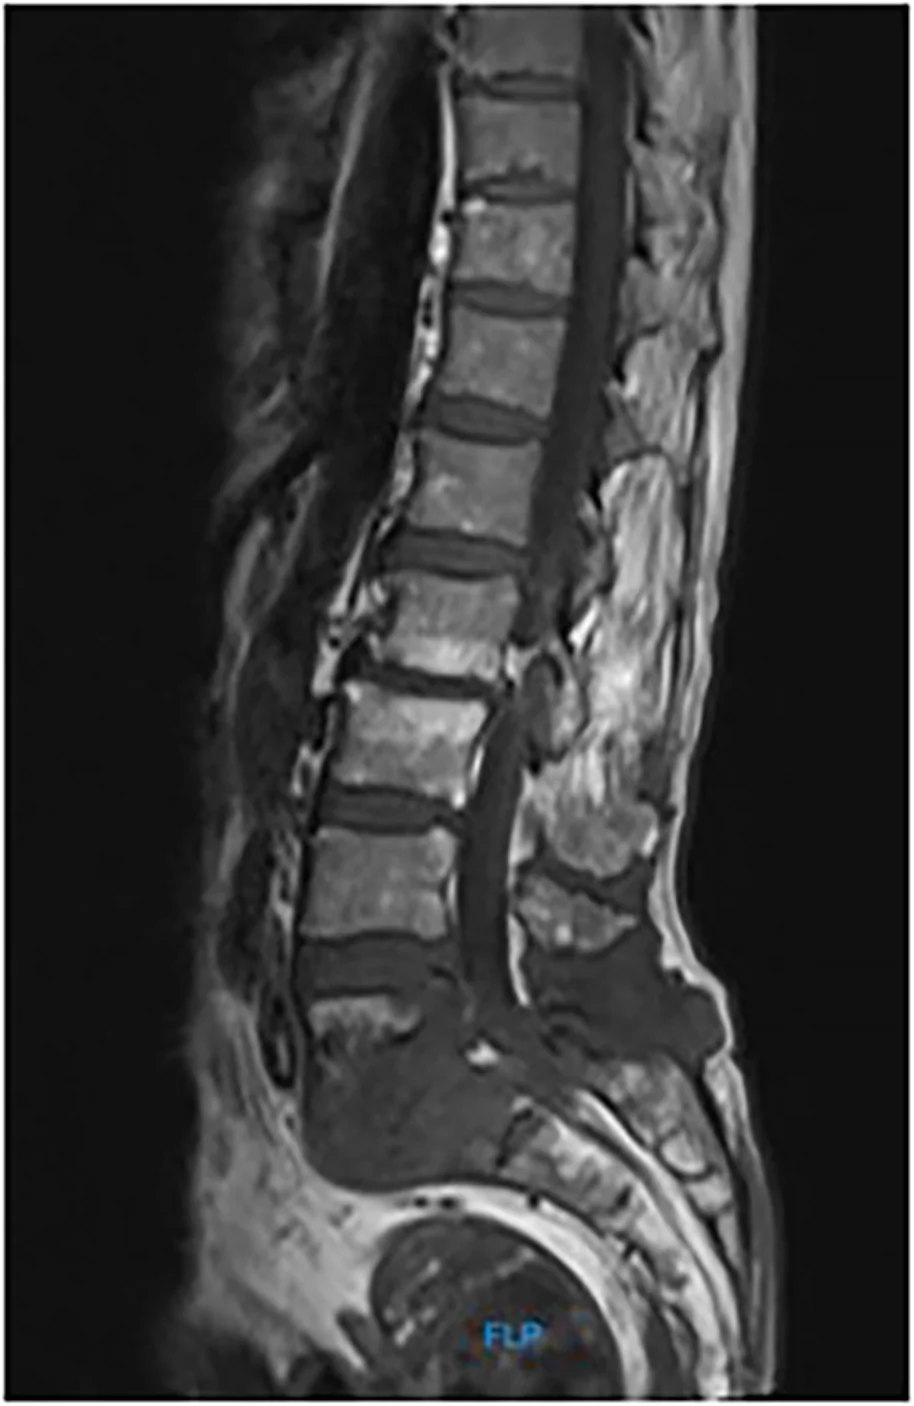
Non‐contrast lumbosacral MRI demonstrating bone marrow edema involving the L5, S1, and S2 vertebral bodies, with destruction of the L5–S1 endplates and associated discitis. The inflammatory process extends to the posterior spinal elements and adjucent paravertebral muscles.
